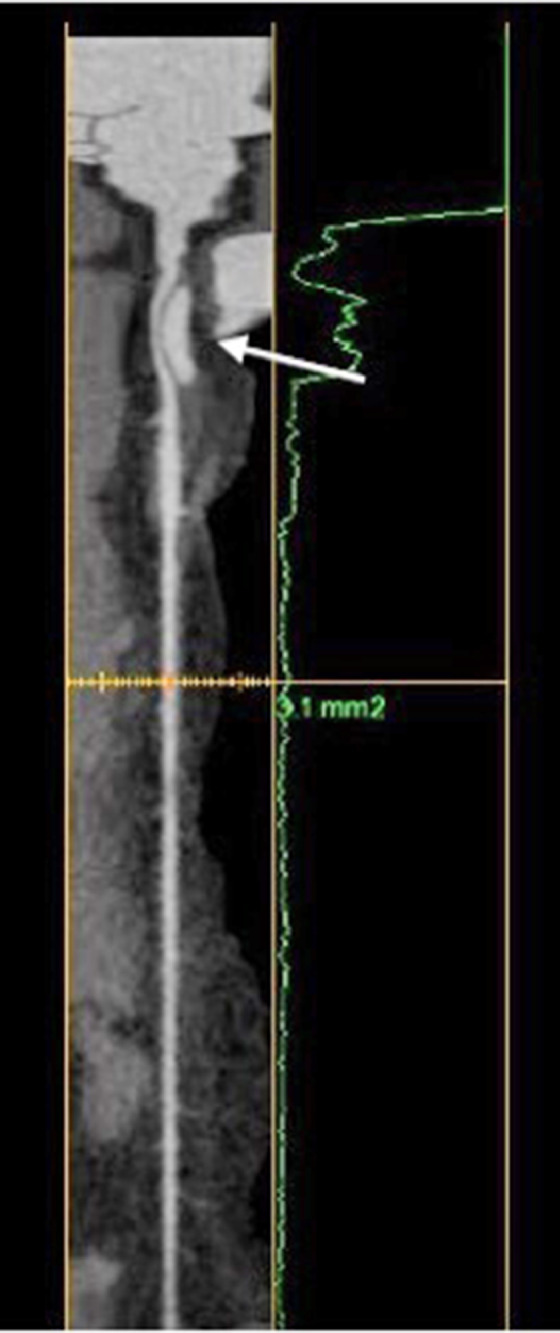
Lumen reconstruction of enhanced CT scan showing the left anterior descending artery in its entire length. The white arrow indicates the dissected tract.
